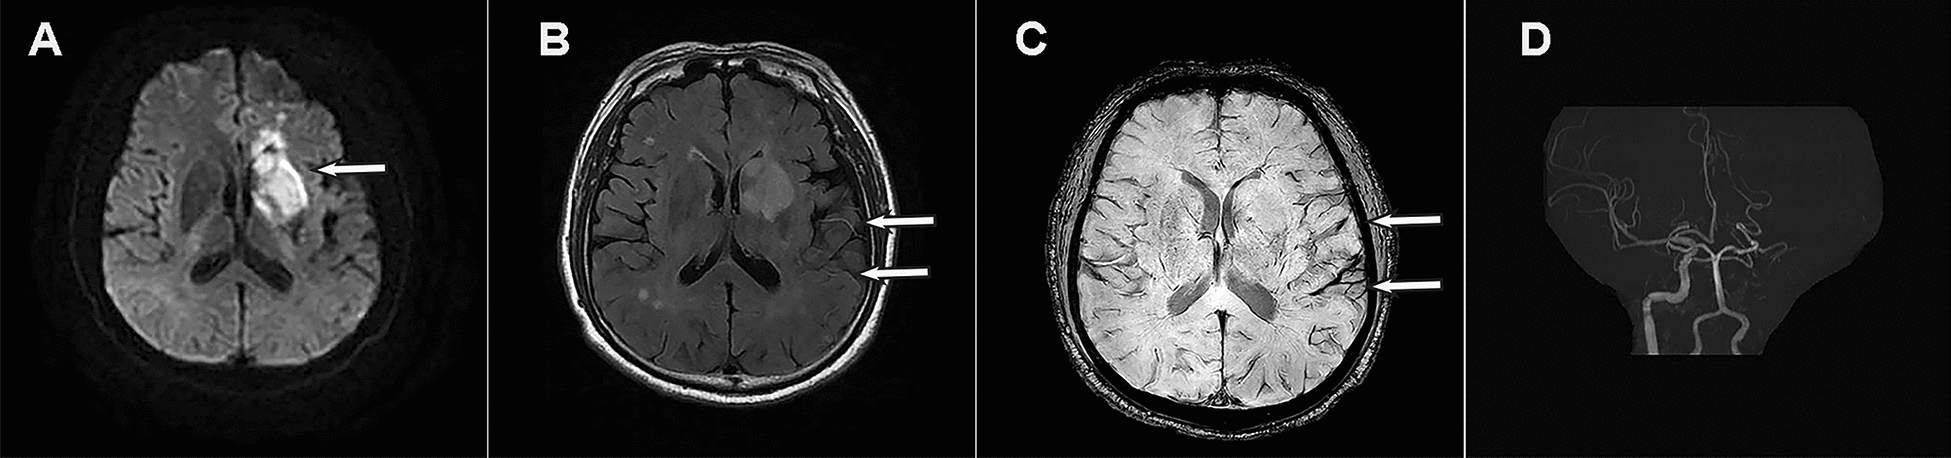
Presentation of a typical patient. A 66-year-old man with left limb weakness was admitted to the hospital, with a 30-h between onset to MR examination. A DWI showed acute infarction; B FLIAR image demonstrated vascular hyperintensity (FVH); C SWI indicated asymmetrical prominent veins sign (APVS); D MRA demonstrated disappearance of left ICA and MCA signals
